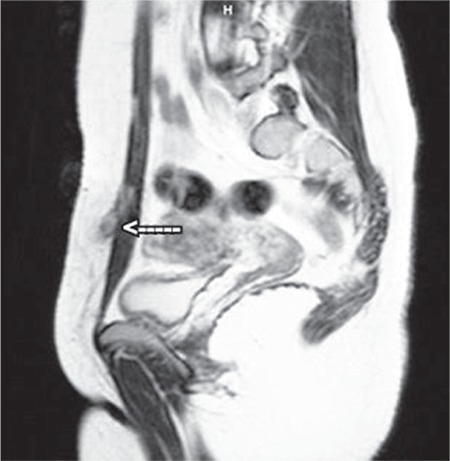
Magnetic resonance image coronar
